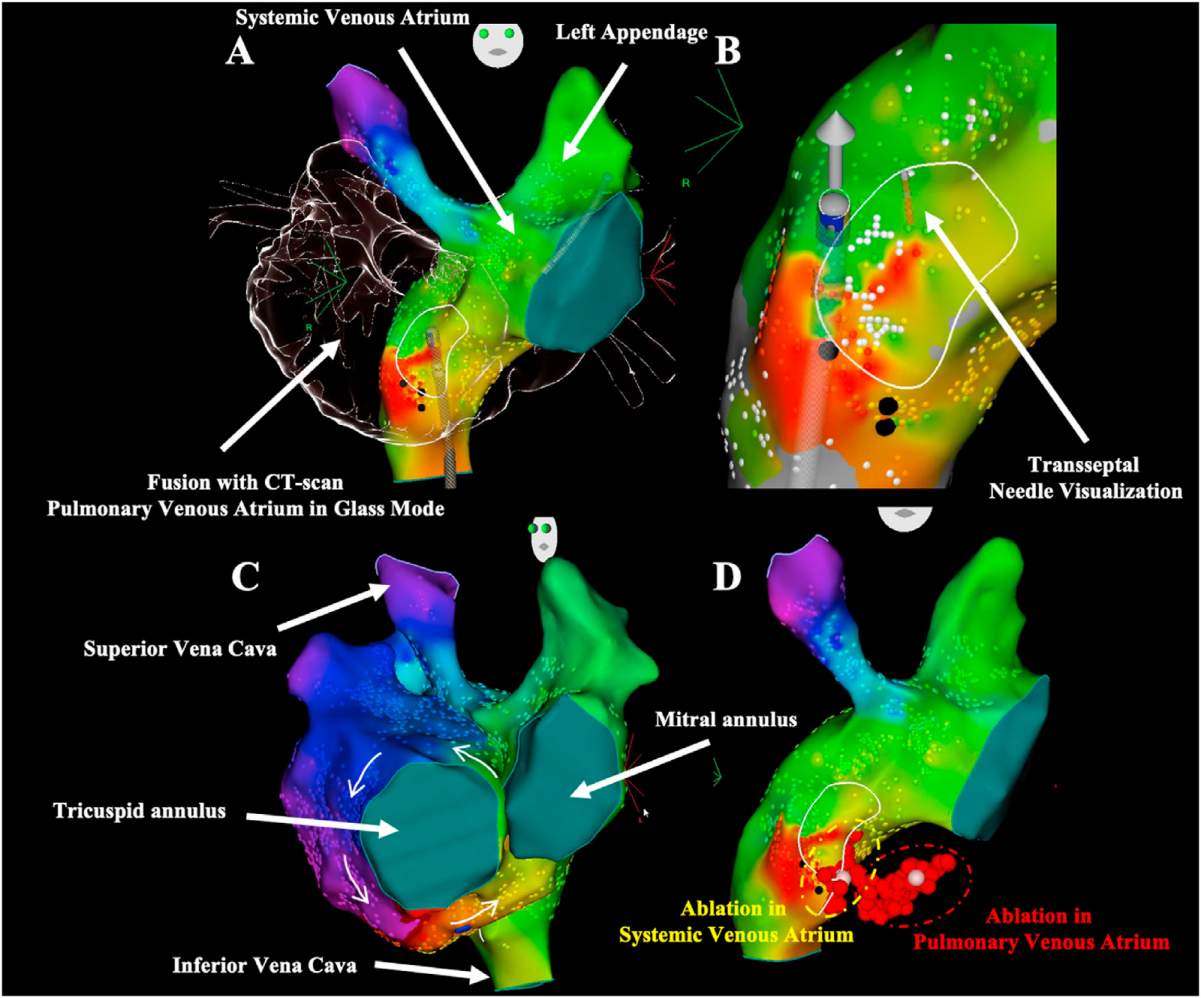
Transbaffle puncture guided by 3D anatomical mapping and cavotricuspid isthmus ablation in a patient with D-TGA and atrial switch operation. A: 3D anatomical reconstruction of the systemic venous atrium (subpulmonary) and fusion with the computed tomography (CT) scan. Note that a reference catheter is positionned in the left atrial appendage directly from the inferior vena cava in the systemic venous atrium B: The optimal site for transbaffle puncture is located and the transseptal needle is advanced and localized by the CARTO® system C: The final activation mapping identifies an atrial flutter around the tricuspid annulus D: Ablation (red dots) in both atrium (on each side of the baffle).
